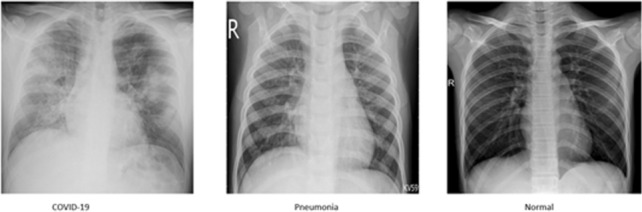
Samples of chest X-ray images from COVID-19 data set
